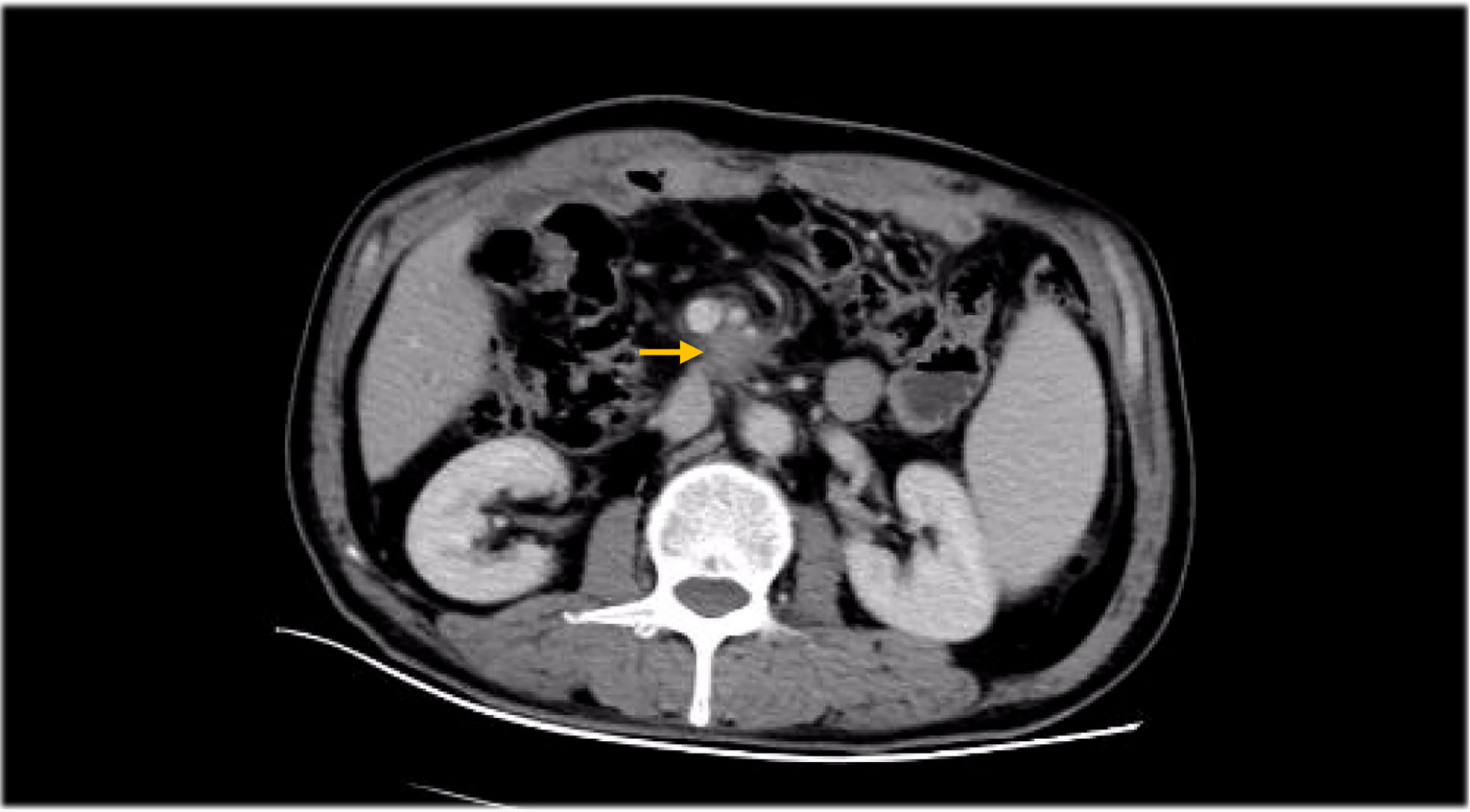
CT Before radiotherapy (abdominal metastatic lymph nodes).
